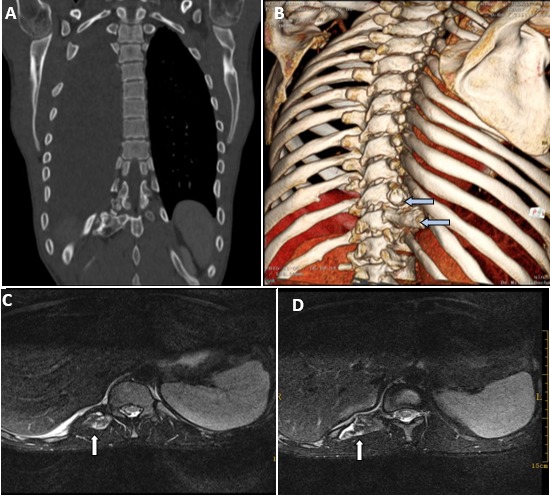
(A) computed tomography of the chest, coronal view with 3-dimension reconstruction; (B) presence of two focal bony masses arising from the right transverse processes of thoracic vertebrae 10 and 11; (C) magnetic resonance imaging of the spine, T2-weighted sequence, axial section, shows two vertebral exostoses arising from the right transverse processes of thoracic vertebrae 10 and 11; (D) measuring 20 × 12 mm and 35 × 25 mm, respectively. That of the transverse process of T11 encroaches on the 11^th^ intercostal space
